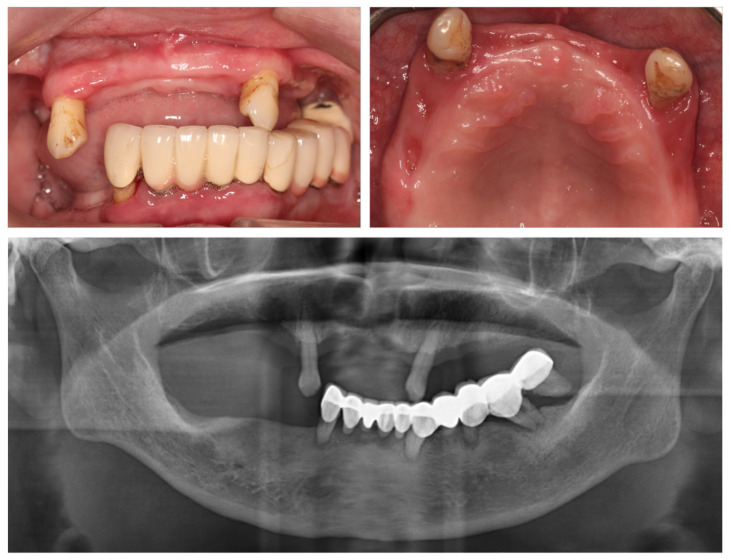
Initial dental status and its panoramic radiograph.
